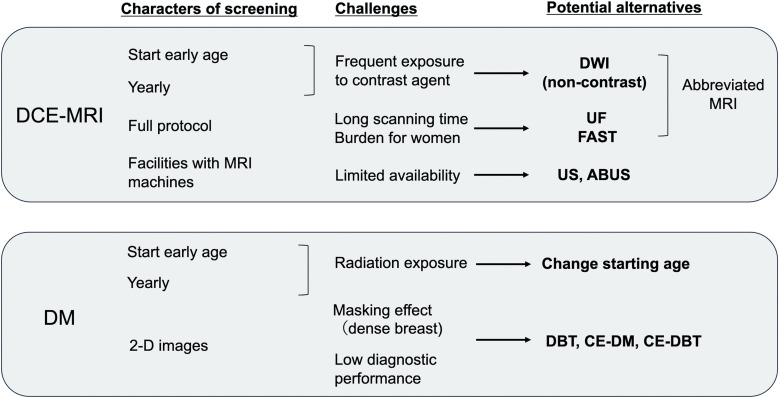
Challenges of standard high-risk screening modalities (DCE-MRI and DM) and proposal of potential alternative modalities. ABUS, automated whole breast ultrasound; CE-DBT, contrast-enhanced digital breast tomosynthesis; CE-DM, contrast-enhanced digital mammography; DBT, digital breast tomosynthesis; DCE-MRI, dynamic contrast-enhanced MRI; DM, digital mammography; DWI, diffusion-weighted image; FAST, first postcontrast subtracted; UF, ultrafast; US, ultrasound.
